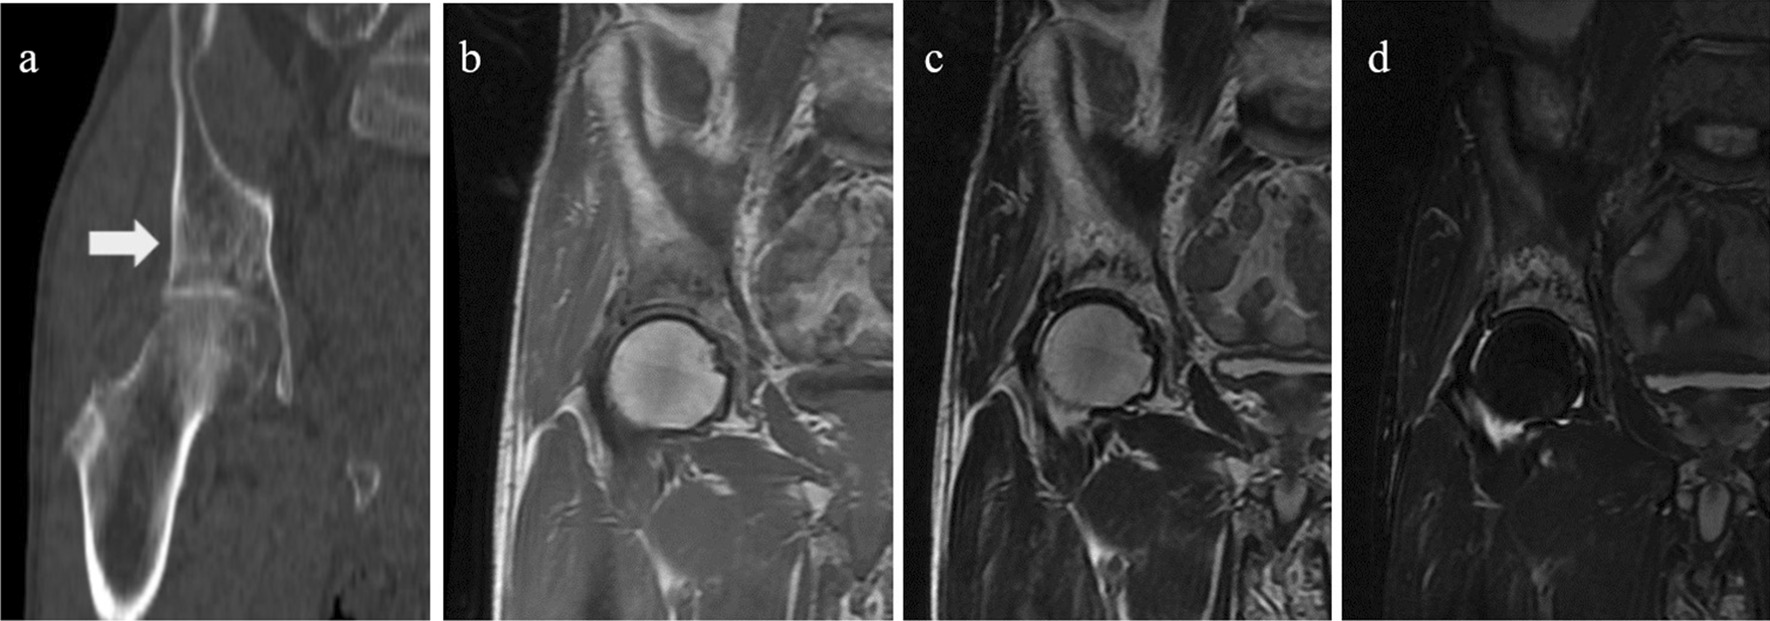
a Computed tomography scan showed evidence of sclerosis with a periosteal reaction in right supra-acetabulum. b Magnetic resonance imaging with T1 weighting showed a linear low signal and an equivalent signal surrounding it at the right supra-acetabulum. c The linear low signal and high signal surrounding it were seen on the T2-weighted image. d The lesion was not suppressed by short TI inversion recovery. The arrow show the site of the lesion
